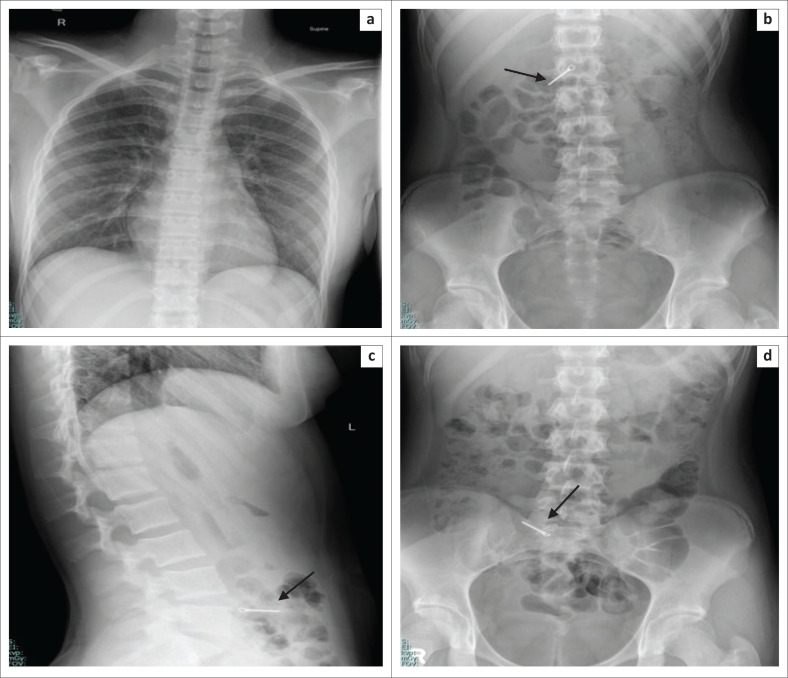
Serial radiographs of a 14-year-old-female patient who accidentally swallowed a loose metallic fragment (arrow) from her dental braces. The foreign body had been transmitted from the oesophagus (a) through the gastric pylorus (b), and later into the right lower quadrant (c and d) on follow-up serial radiographs. The patient was managed conservatively without any complications.
